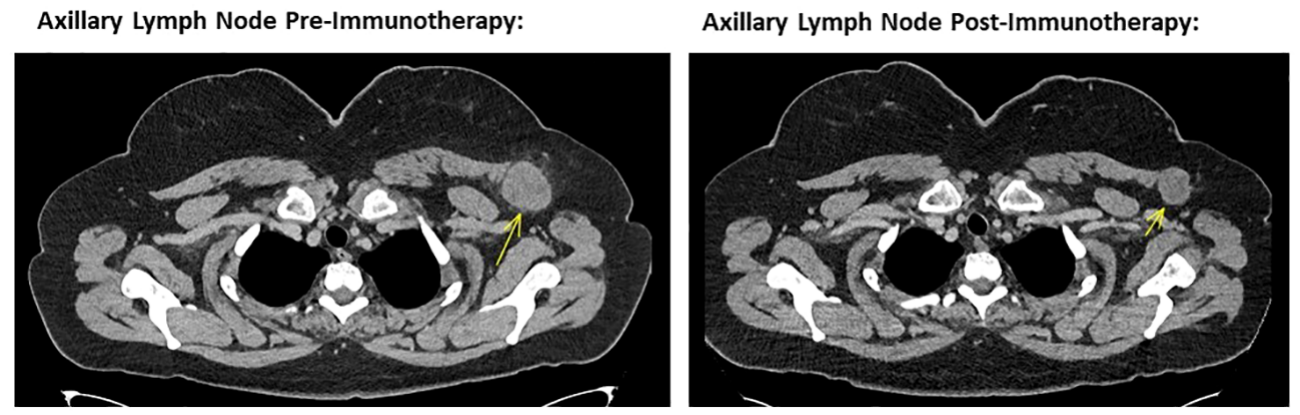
Left image showing cross sectional CT imaging of the head, chest, abdomen and pelvis in November 2022, identifying a 40mm left axillary lesion compatible with a melanoma metastasis. Right image showing cross sectional CT imaging of the head, chest, abdomen and pelvis from October 2023, noting the same left axillary lesion measuring 28 mm in short axis. This is consistent with a marked response to ipilimumab and nivolumab combination immunotherapy.
